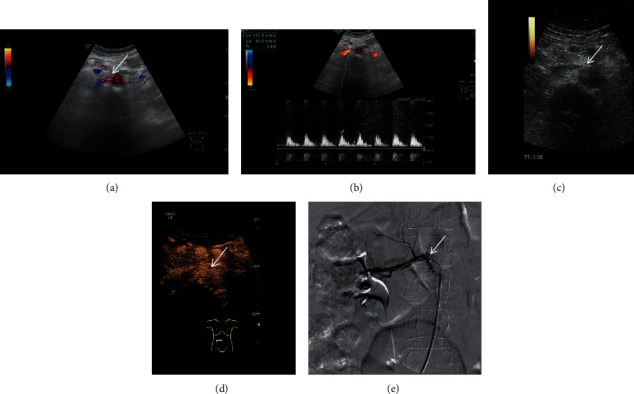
DUS images, CEUS images, and their corresponding DSA images of grade I (stenosis rate: 30%-49%). (a) Color Doppler ultrasound was used to show the long axis section of renal artery. (b) The peak systolic velocity at the stenosed segment of the renal artery was obtained by pulse Doppler ultrasound. (c) Two-dimensional grayscale ultrasound cannot clearly show renal artery stenosis. (d) Contrast-enhanced ultrasound can increase the visualization of renal artery and can be used for the display of grade I renal artery stenosis. (e) Digital subtraction angiography confirms the result of contrast-enhanced ultrasound and shows the diameter of the main renal artery decreased at the stenosed segment in the patient with renal artery stenosis.
